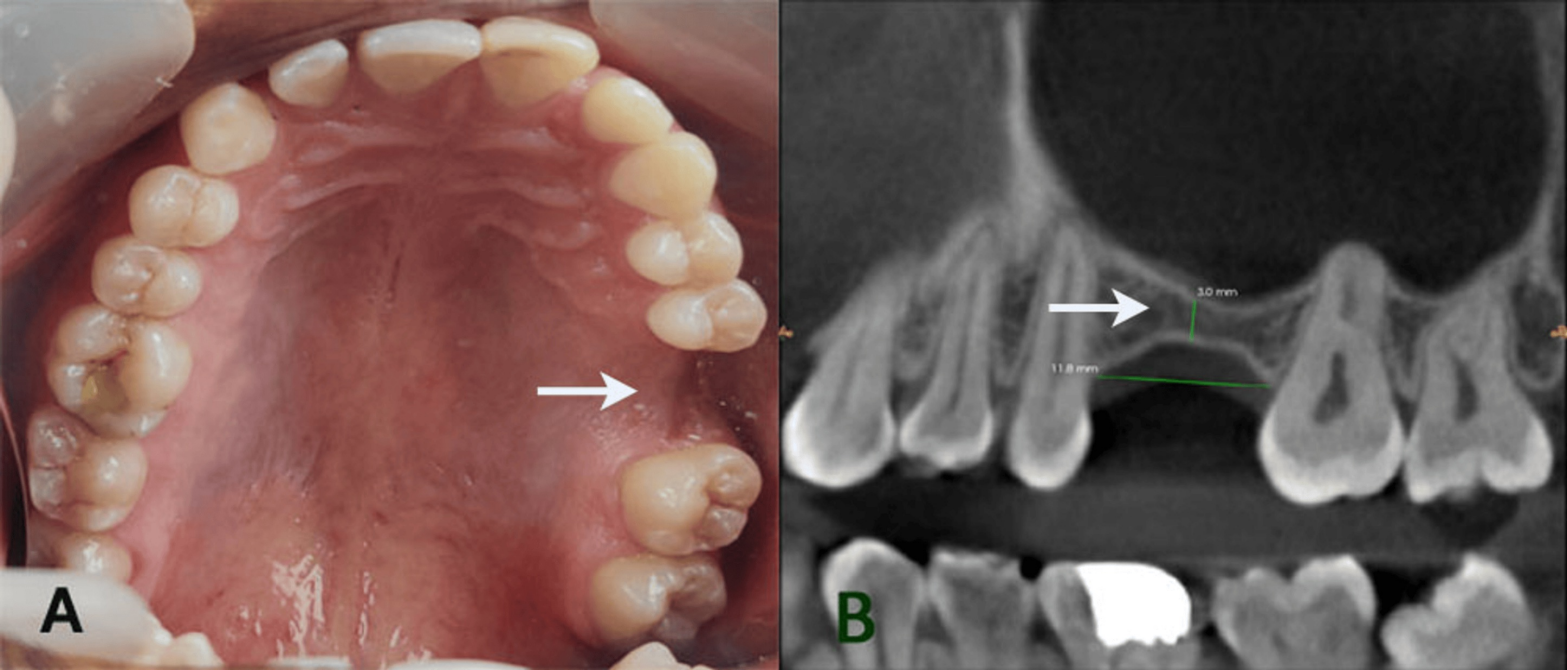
Intraoral view of missing #26 (A) and preoperative CBCT showing the alveolar height of 3 mm and width of 11.8 mm in the area of #26 (B) CBCT: cone beam computed tomography
